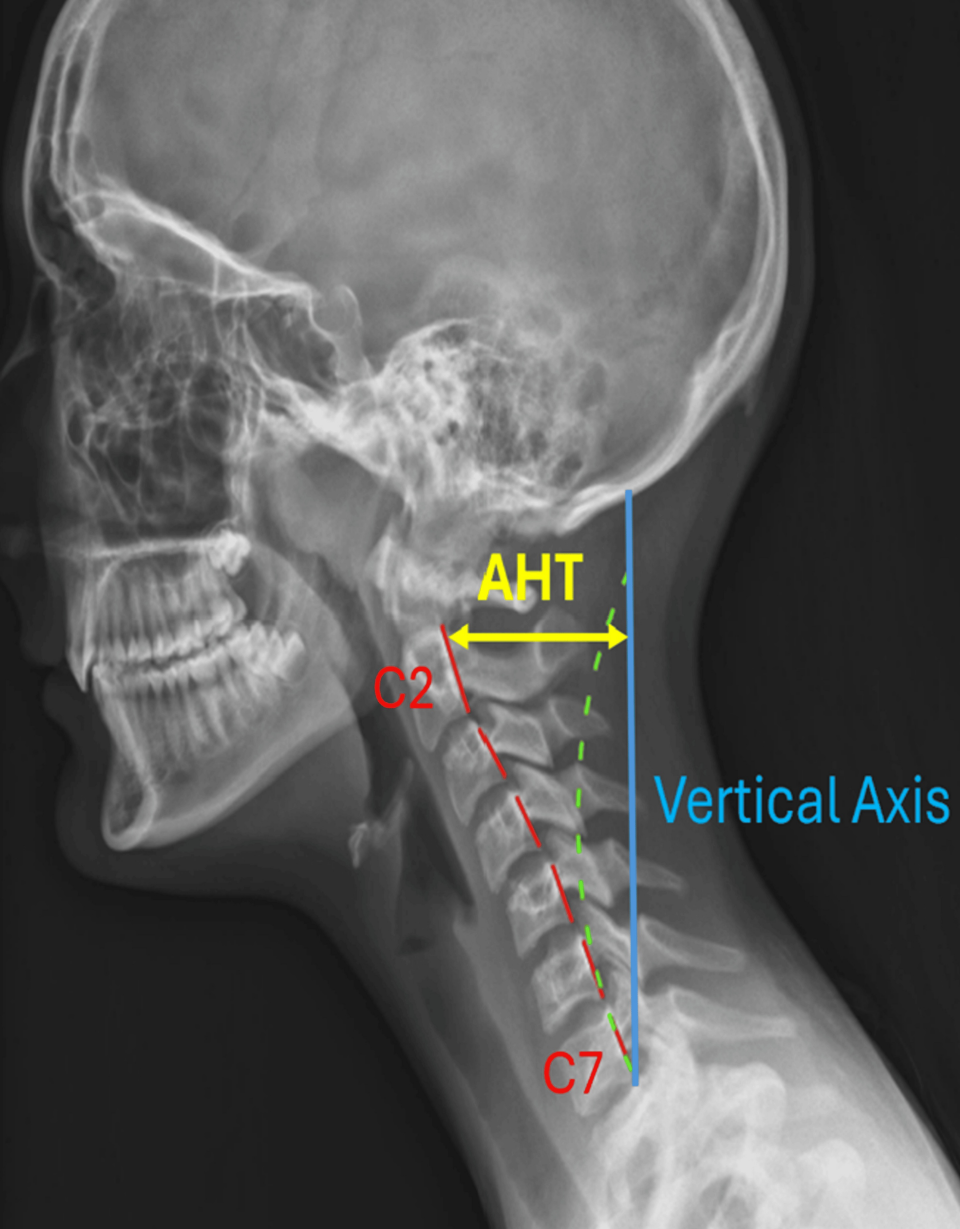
Sagittal view of a lateral neutral cervical radiograph. The green dotted line indicates the estimated normal spinal alignment and the expected path of the posterior longitudinal ligament, while the red line represents the patient's actual spinal alignment and corresponding ligament path. The blue line is a vertical line drawn from the posterior-inferior endplate of C7. The yellow line represents AHT, measured from the posterior-superior endplate of C2 to the vertical axis extending from C7 AHT: anterior head translation
